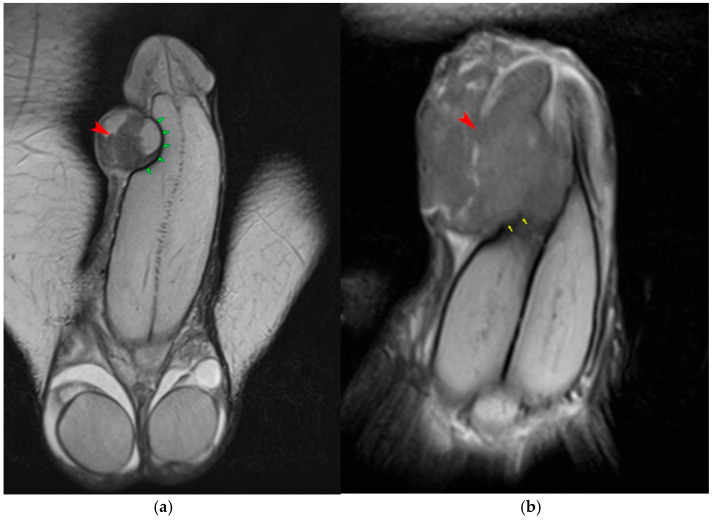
Squamous cell carcinoma of the penis in two different patients. (a) Coronal T2-weighted image shows a penile mass (red arrowhead) without invasion of the corpora cavernosa; the tunica albuginea (green arrows) remains intact. (b) In another patient, the tumor (red arrowhead) infiltrates the corpora cavernosa, evidenced by disruption of the tunica albuginea (yellow arrows).
